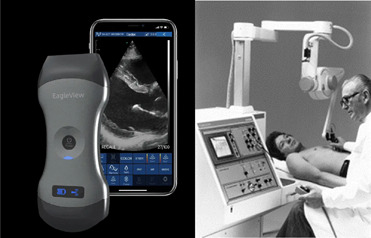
A wireless probe ultrasound scanner 2024 and a scanner from 1977.
